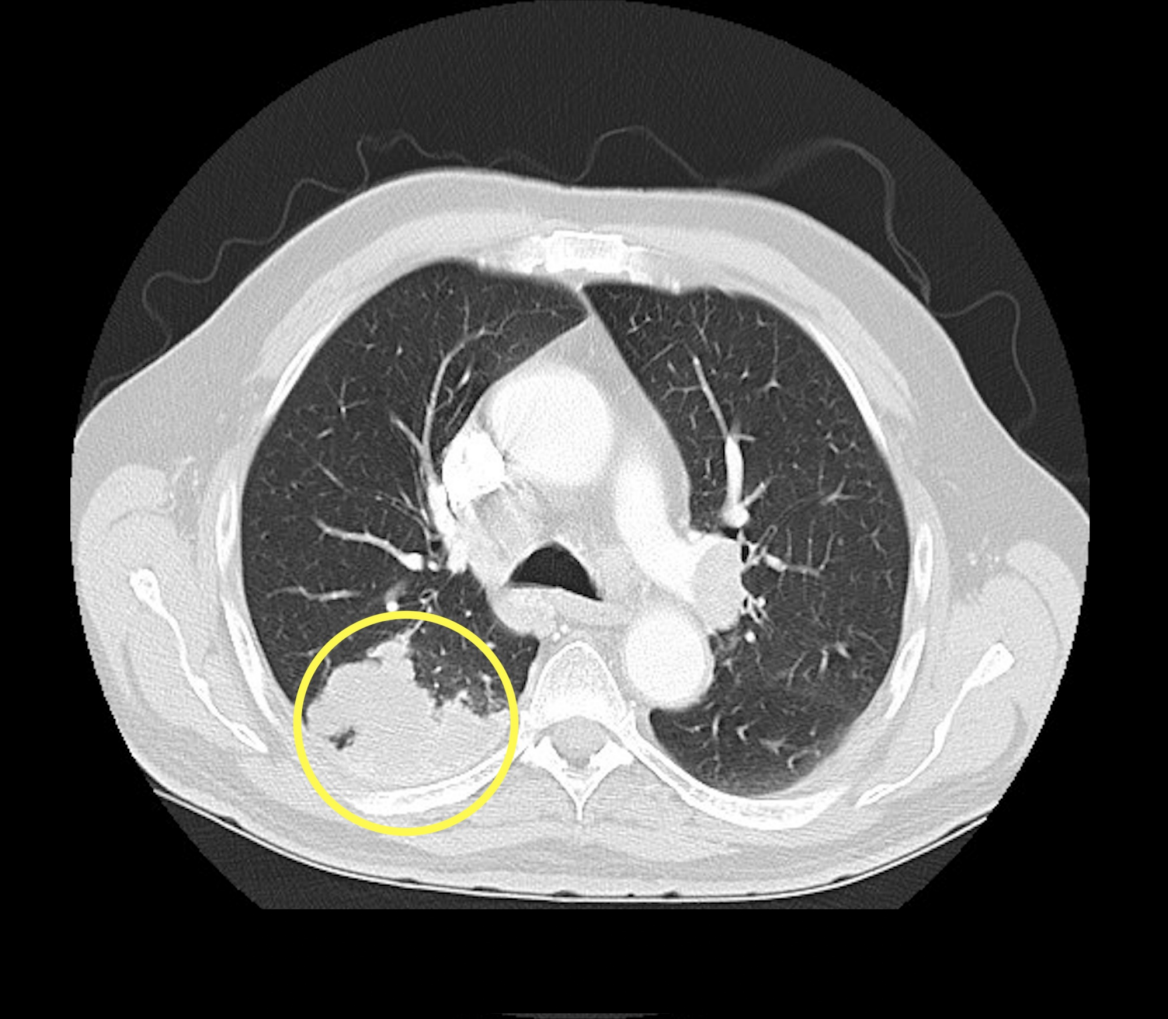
Axial contrast enhanced computed tomography (CT) chest (lung window) demonstrating a lobulated pleural-based mass in the poster segment of the right upper lobe (yellow circle).
